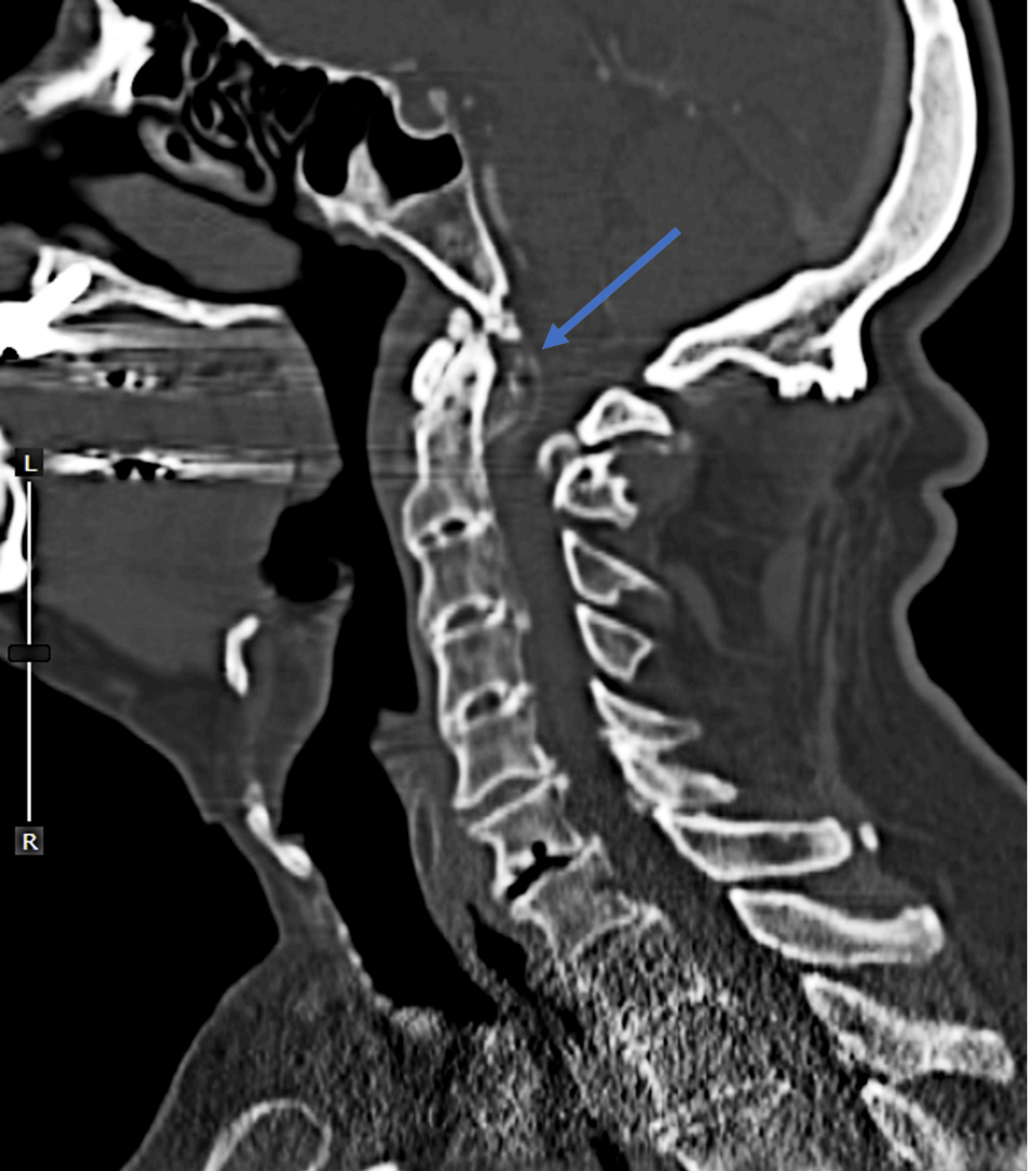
Cervical Spine CTA Midsagittal plane CTA of the cervical spine demonstrating a retrodental calcified compressive mass (blue arrow).
